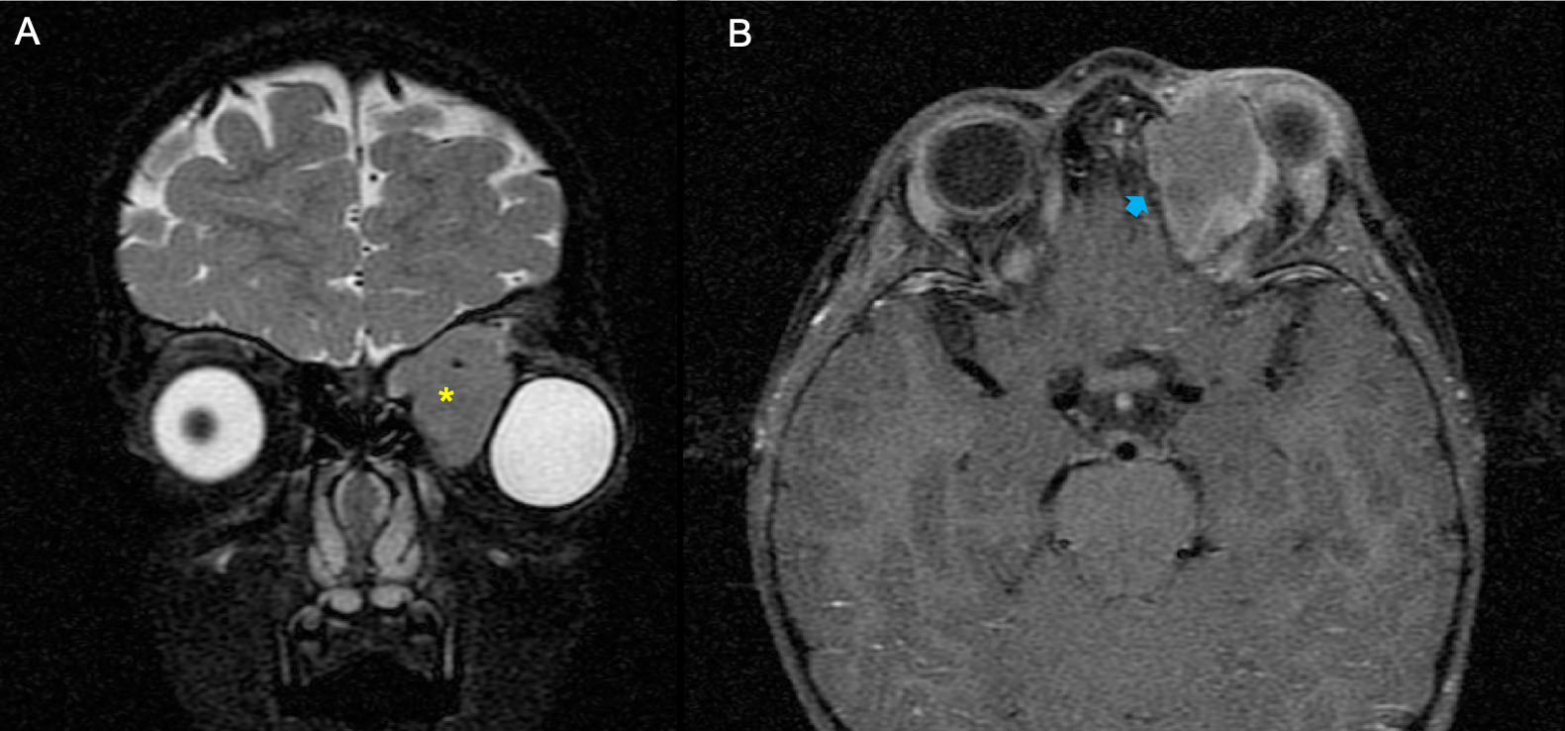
Example of a patient with orbital rhabdomyosarcoma (RMS) and extensive bone erosion (BE). (A, B) MRI showed left orbital mass on the coronal plane fat-saturated T2-weighted image (*yellow asterisk*) (A) and an extensive grade of BE on the axial T1 fat-saturated post-contrast sequence (*blue arrow*) (B).
